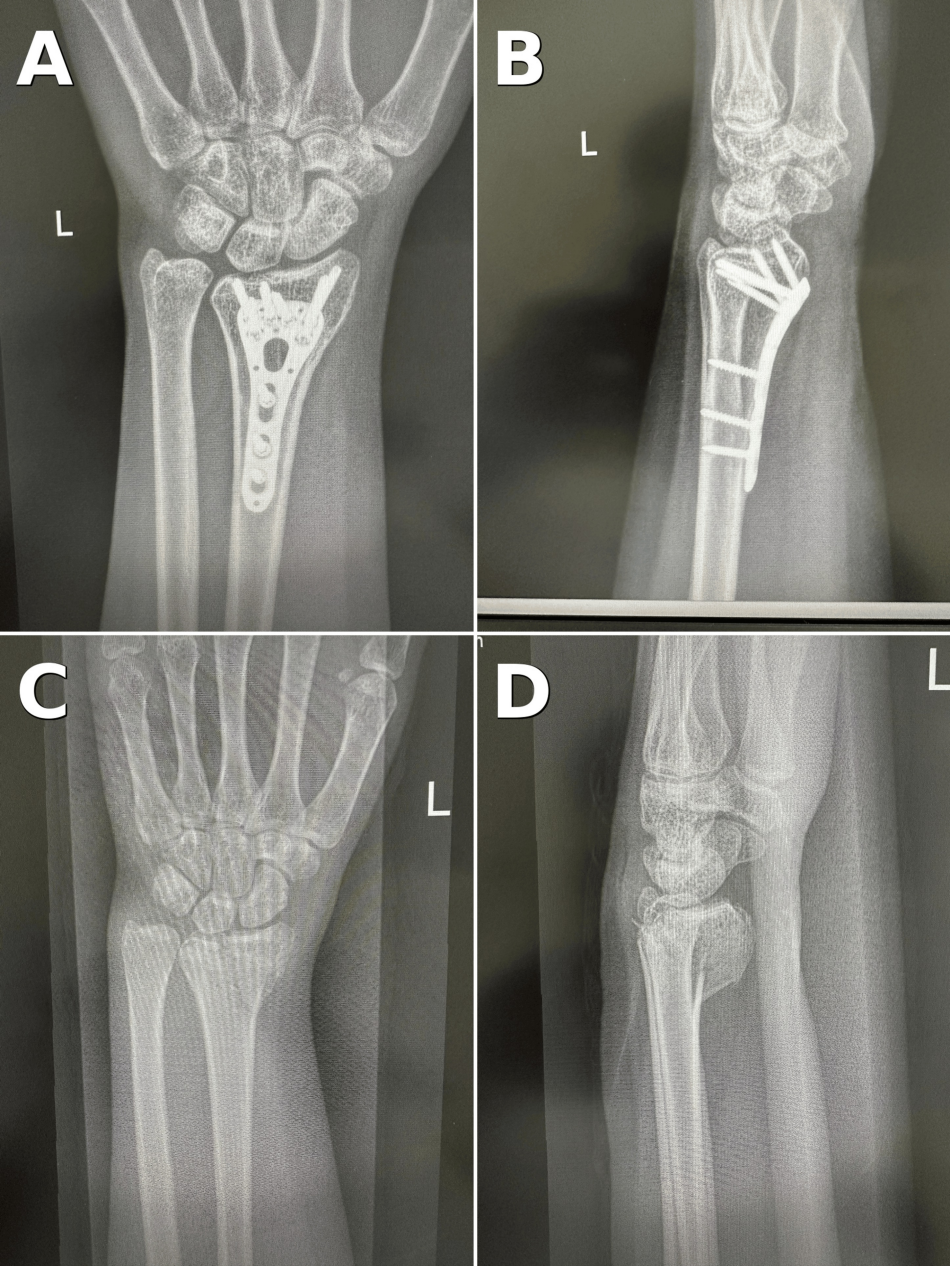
Pre- and postoperative radiographs of an unstable distal radius fracture treated with volar locking plate fixation (A, B) Immediate postoperative posteroanterior and lateral views of the left wrist showing restored radial height, radial inclination, and volar tilt with a volar locking plate positioned proximal to the watershed line.
